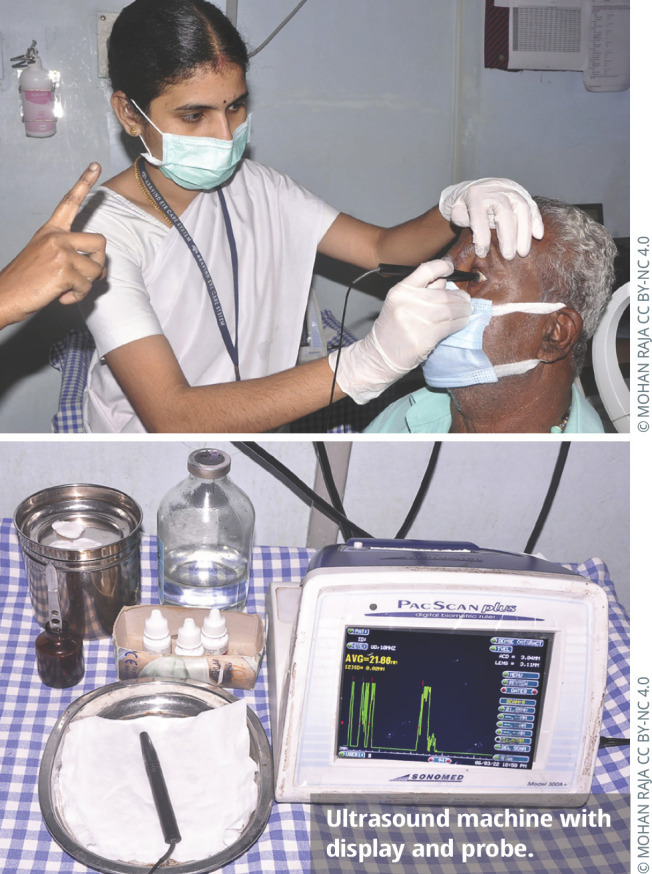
A biometrist performs contact (applanation) ultrasound biometry. INDIA
